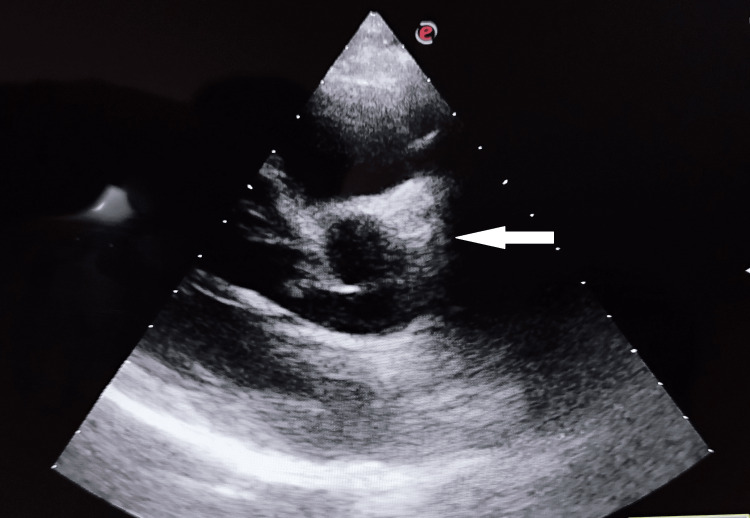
Echocardiography demonstrating vegetation on the aortic valve
